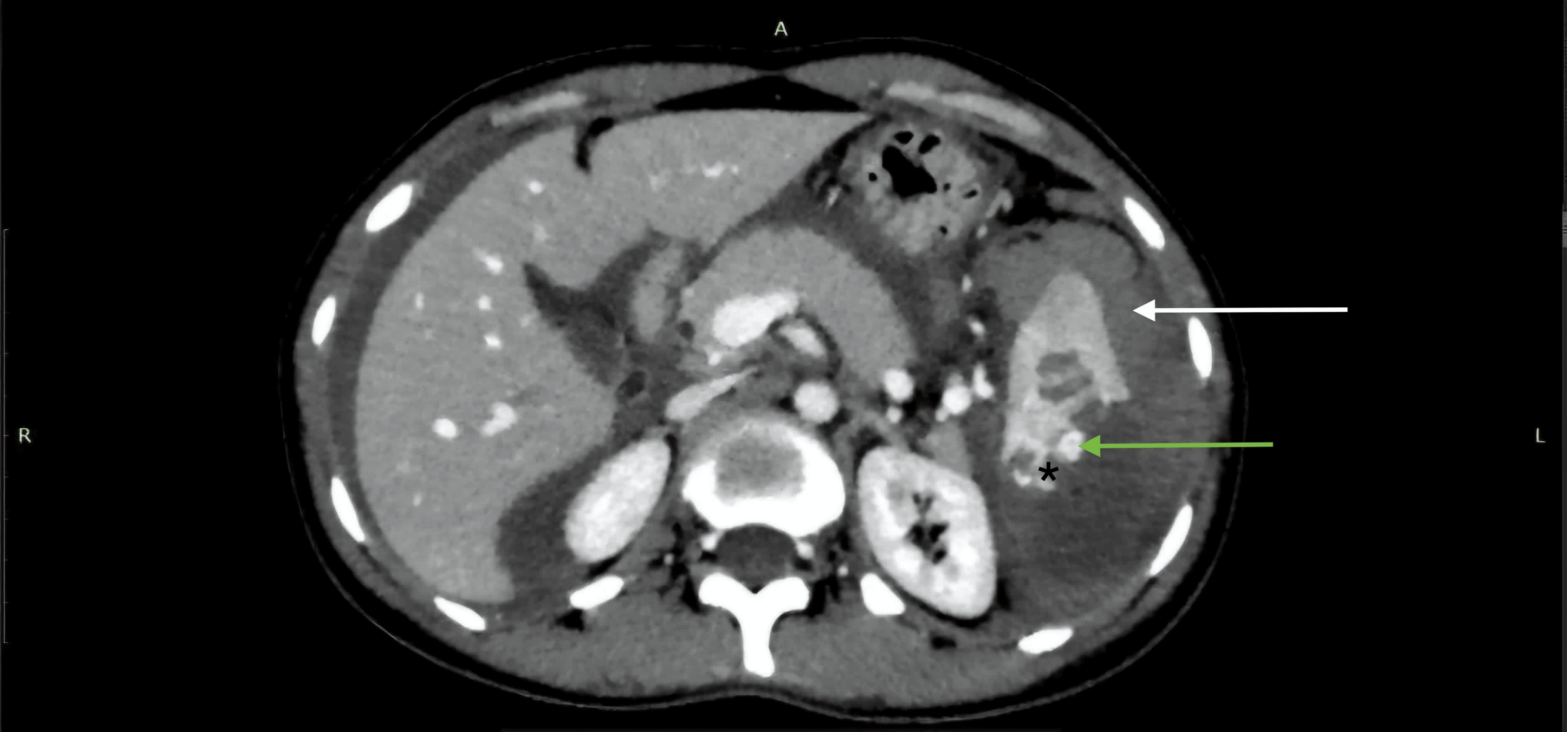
AAST grade IV splenic injury with parenchymal laceration, devascularization, and subcapsular hematoma on CECT (PV protocol). A 31-year-old female with a history of RTA underwent CECT of the abdomen using the PV protocol. The axial CECT image shows a massive parenchymal laceration with approximately 40% devascularization of the splenic parenchyma (black star), a subcapsular hematoma (white arrow), and a small focus of active contrast extravasation (green arrow) confined within the splenic capsule. Findings were consistent with the AAST grade IV splenic injury. AAST: American Association for the Surgery of Trauma; CECT: contrast-enhanced computed tomography; PV: portal venous; RTA: road traffic accident.
